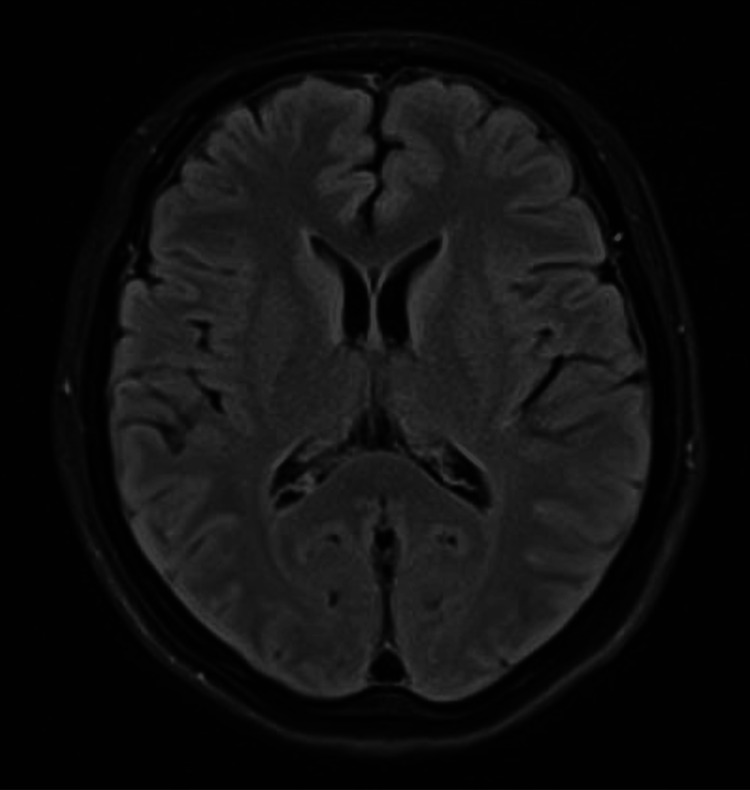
Contrasted MRI brain showed no evidence of intracranial pathology, such as infarct, hemorrhage, mass lesion, or parenchymal enhancing lesion. The ventricles, sulci, and cisterns are age-appropriate in size and configuration. The basal cisterns are preserved MRI: magnetic resonance imaging
